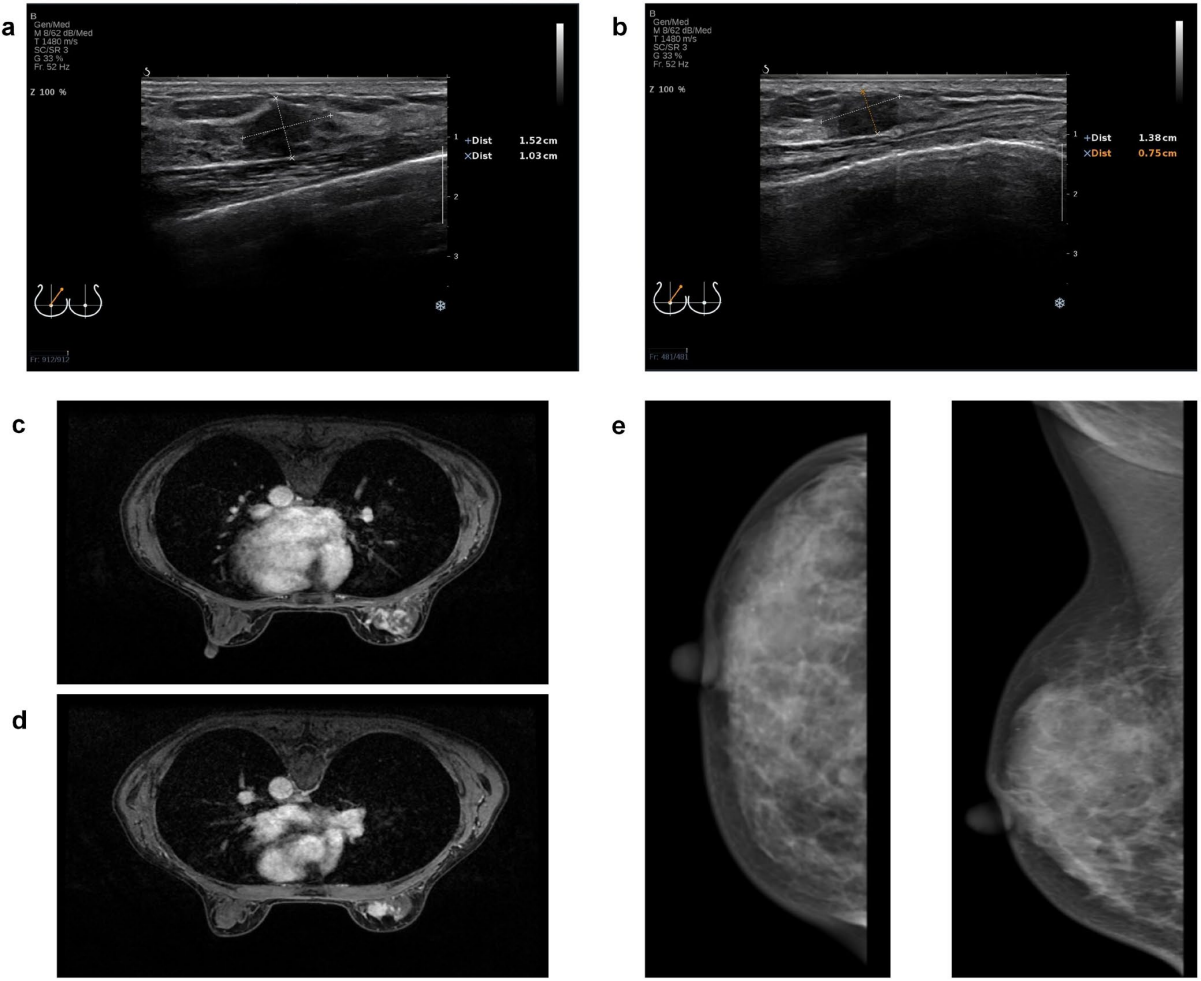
Diagnostic imaging findings. (a, b) Ultrasound images of the affected breast. (c, d) Contrast‐enhanced breast magnetic resonance imaging (MRI). (e) Mammographic images of the affected breast (craniocaudal and mediolateral oblique views).
